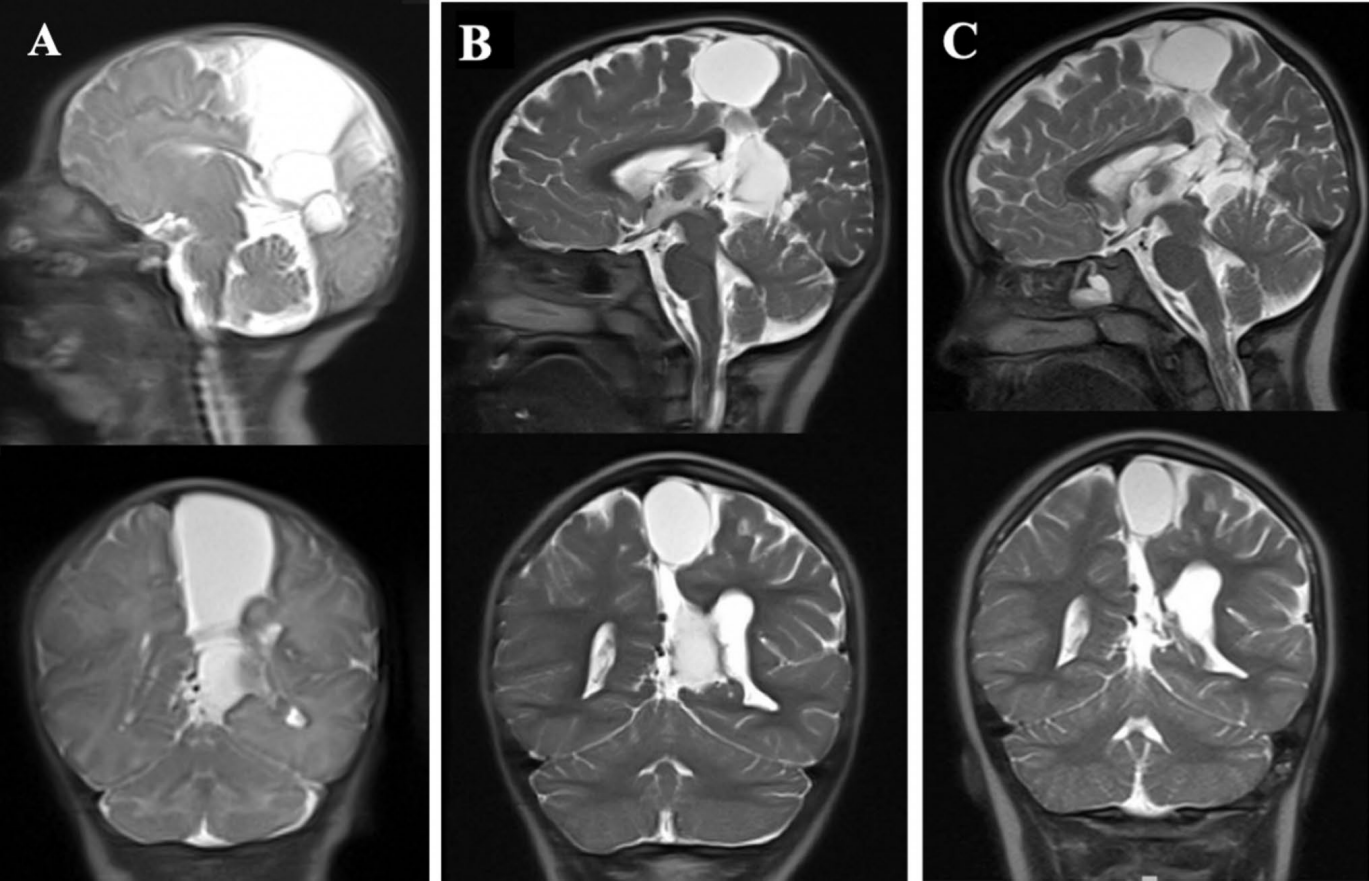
A Sagittal (upper) and coronal (lower) brain fast sequence magnetic resonance imaging (MRI) on day 2 of life, demonstrating a large cluster of extra-axial cystic structures at the medial aspect of the left parietal lobe extending inferior to the quadrigeminal cistern with mass effect on the adjacent brain and left lateral ventricle, as well as hypoplastic posterior corpus callosum. B Sagittal (upper) and coronal (lower) brain fast sequence MRI at 6 years of age, showing decrease in size of the cyst. C Sagittal (upper) and coronal (lower) brain fast sequence MRI at 8 years of age, which showed further decrease in size in the cyst
